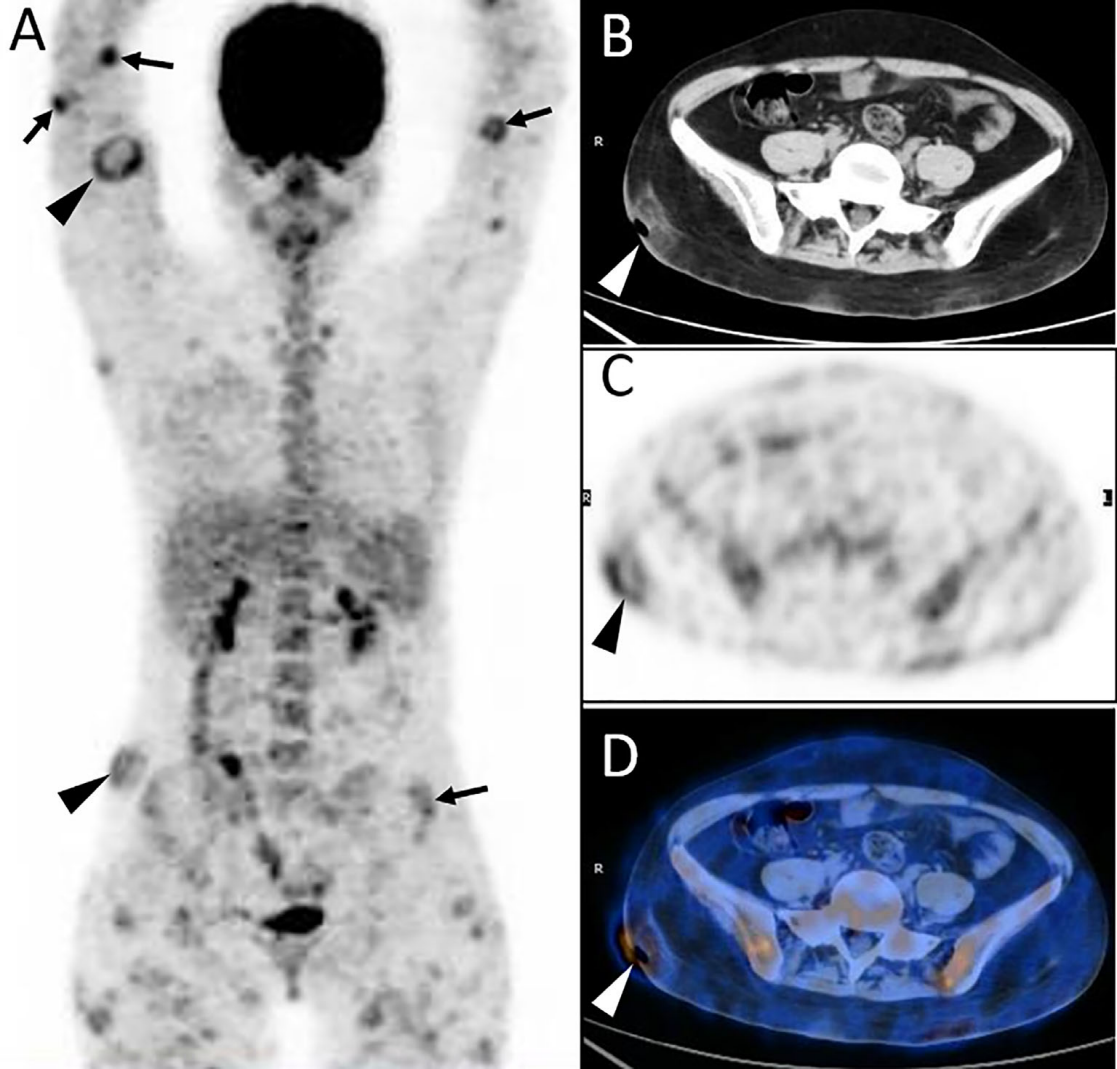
Twenty-year-old female with subcutaneous panniculitis-like T-cell lymphoma (SPTCL). Three-dimensional maximum intensity projection PET image (A) shows multiple nodules and diffuse plaques with high uptake of ^18^F-FDG in both upper arms and legs, lower back, and buttock. Multiple skin ulcerations with a maximum diameter of 10 cm present as circular uptake of ^18^F-FDG (arrowheads) on PET/CT scan. Abdominal axial CT (B), corresponding PET (C), and fusion PET/CT (D) images from ^18^F-FDG PET/CT clearly show the defection of skin in the right hip, which represents ulcer caused by lymphoma involvement. This patient died 4 months after diagnosis of SPTCL.
